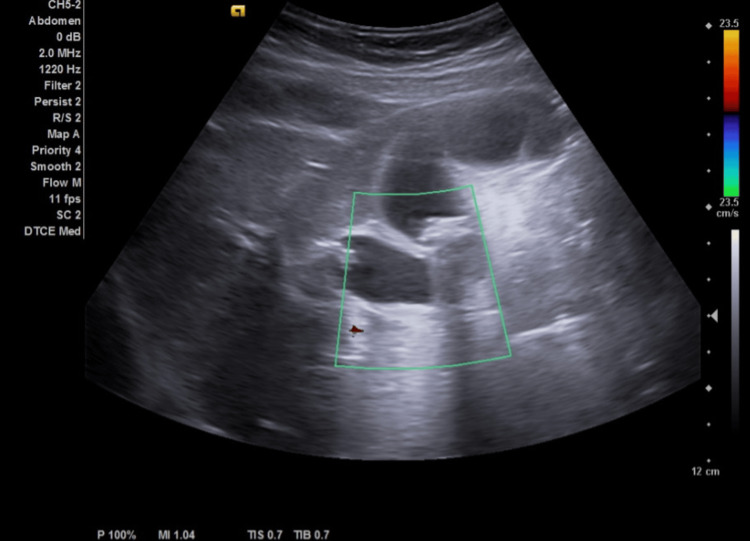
Abdominal ultrasound images of a situs inversus patient with acute cholecystitis. The ultrasound of the 40-year-old female patient indicated gallstones in the gallbladder, with posterior shadowing reflecting the stones.
